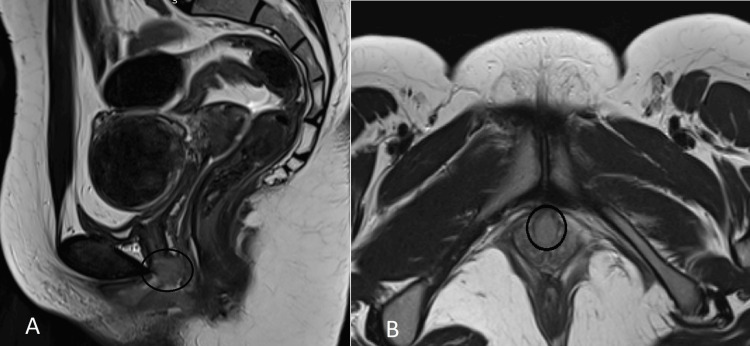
(A) Sagittal T2-weighted image demonstrating a well-defined, enhancing lesion (circled) located in the distal urethra, extending posteriorly to abut the anterior vaginal wall. (B) Axial T2-weighted image showing the same lesion (circled), centered in the distal urethra and predominantly intraluminal, without evidence of deep pelvic extension or invasion into adjacent structures.
